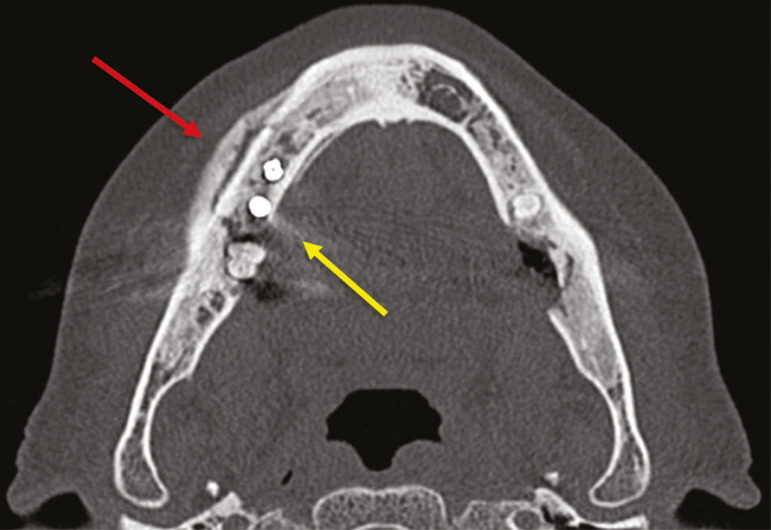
Axial CT of the face showing a periosteal reaction in the right branch of the mandible, together with bone sclerosis (red arrow). Note the areas of erosion areas throughout the lingual cortex on the right (yellow arrow). Taken together, these findings are suggestive of osteomyelitis.
